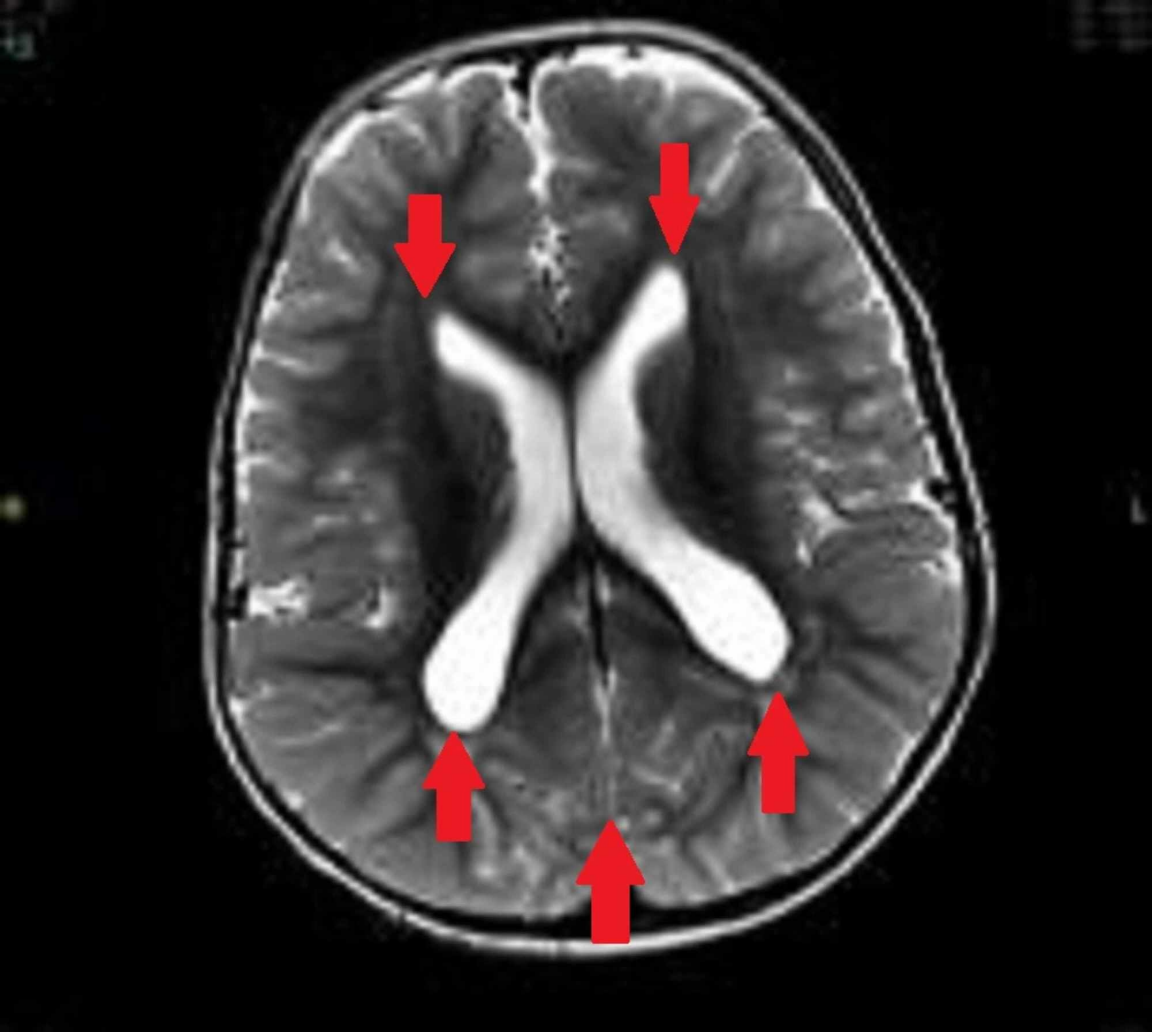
CT scan brain showing megalencephaly with enlarged ventricles and prominent trigone (red arrows).
